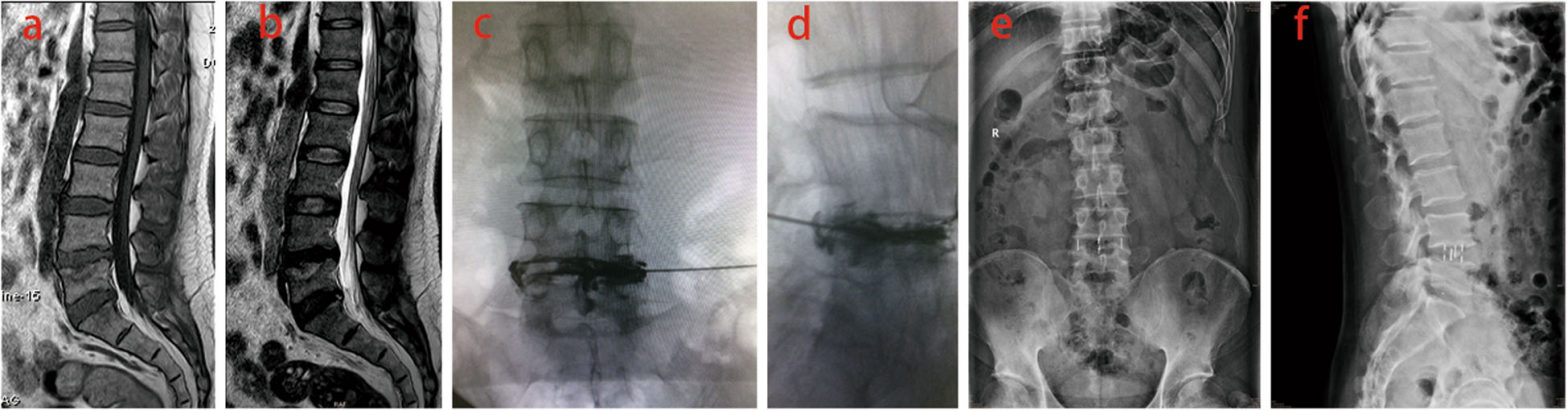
The vertebral body bone marrow surrounding painful Schmorl’s nodes was characterized by low T1 and high T2 signals in MRI (a, b). The patient experienced severe LBP and confirmed to be discogenic by discography (c, d). After the discoblock was administered, the patient complained LBP relieved. The patient had reoccurring pain and underwent OLIF after 8 months follow-up (e, f)
